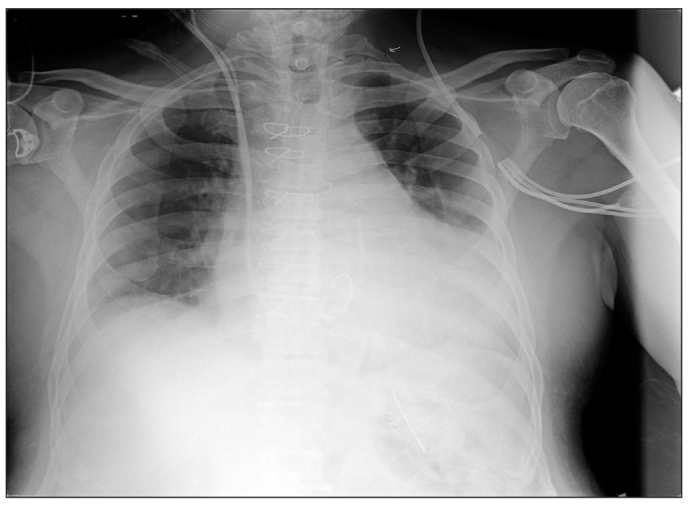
Postoperative chest radiograph showing fracture of left first rib
